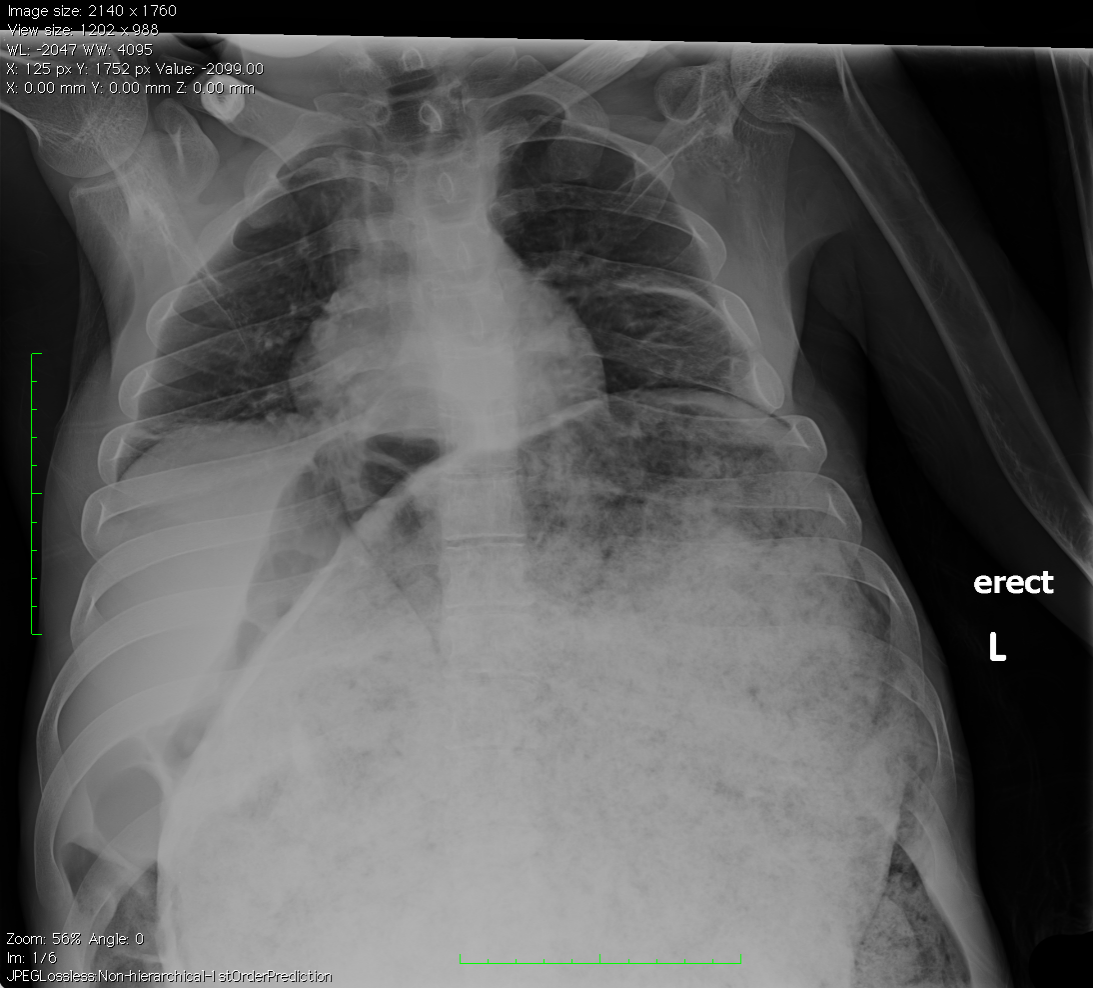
X-ray revealing severe colonic dilatation from the pseudo-obstruction with large stool collection.
